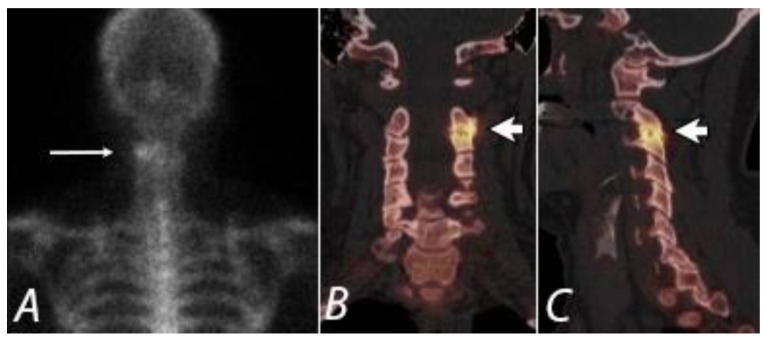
Posterior planar (A) (long white arrow), coronal (B), and sagittal (C) SPECT/CT images from a ^99m^Tc bisphosphonate bone scan of a 43 year old women with left neck pain. Marked radiopharmaceutical uptake corresponds to a severely degenerative left C2/3 facet joint (short white arrows), identifying a potentially targetable pain generator.
